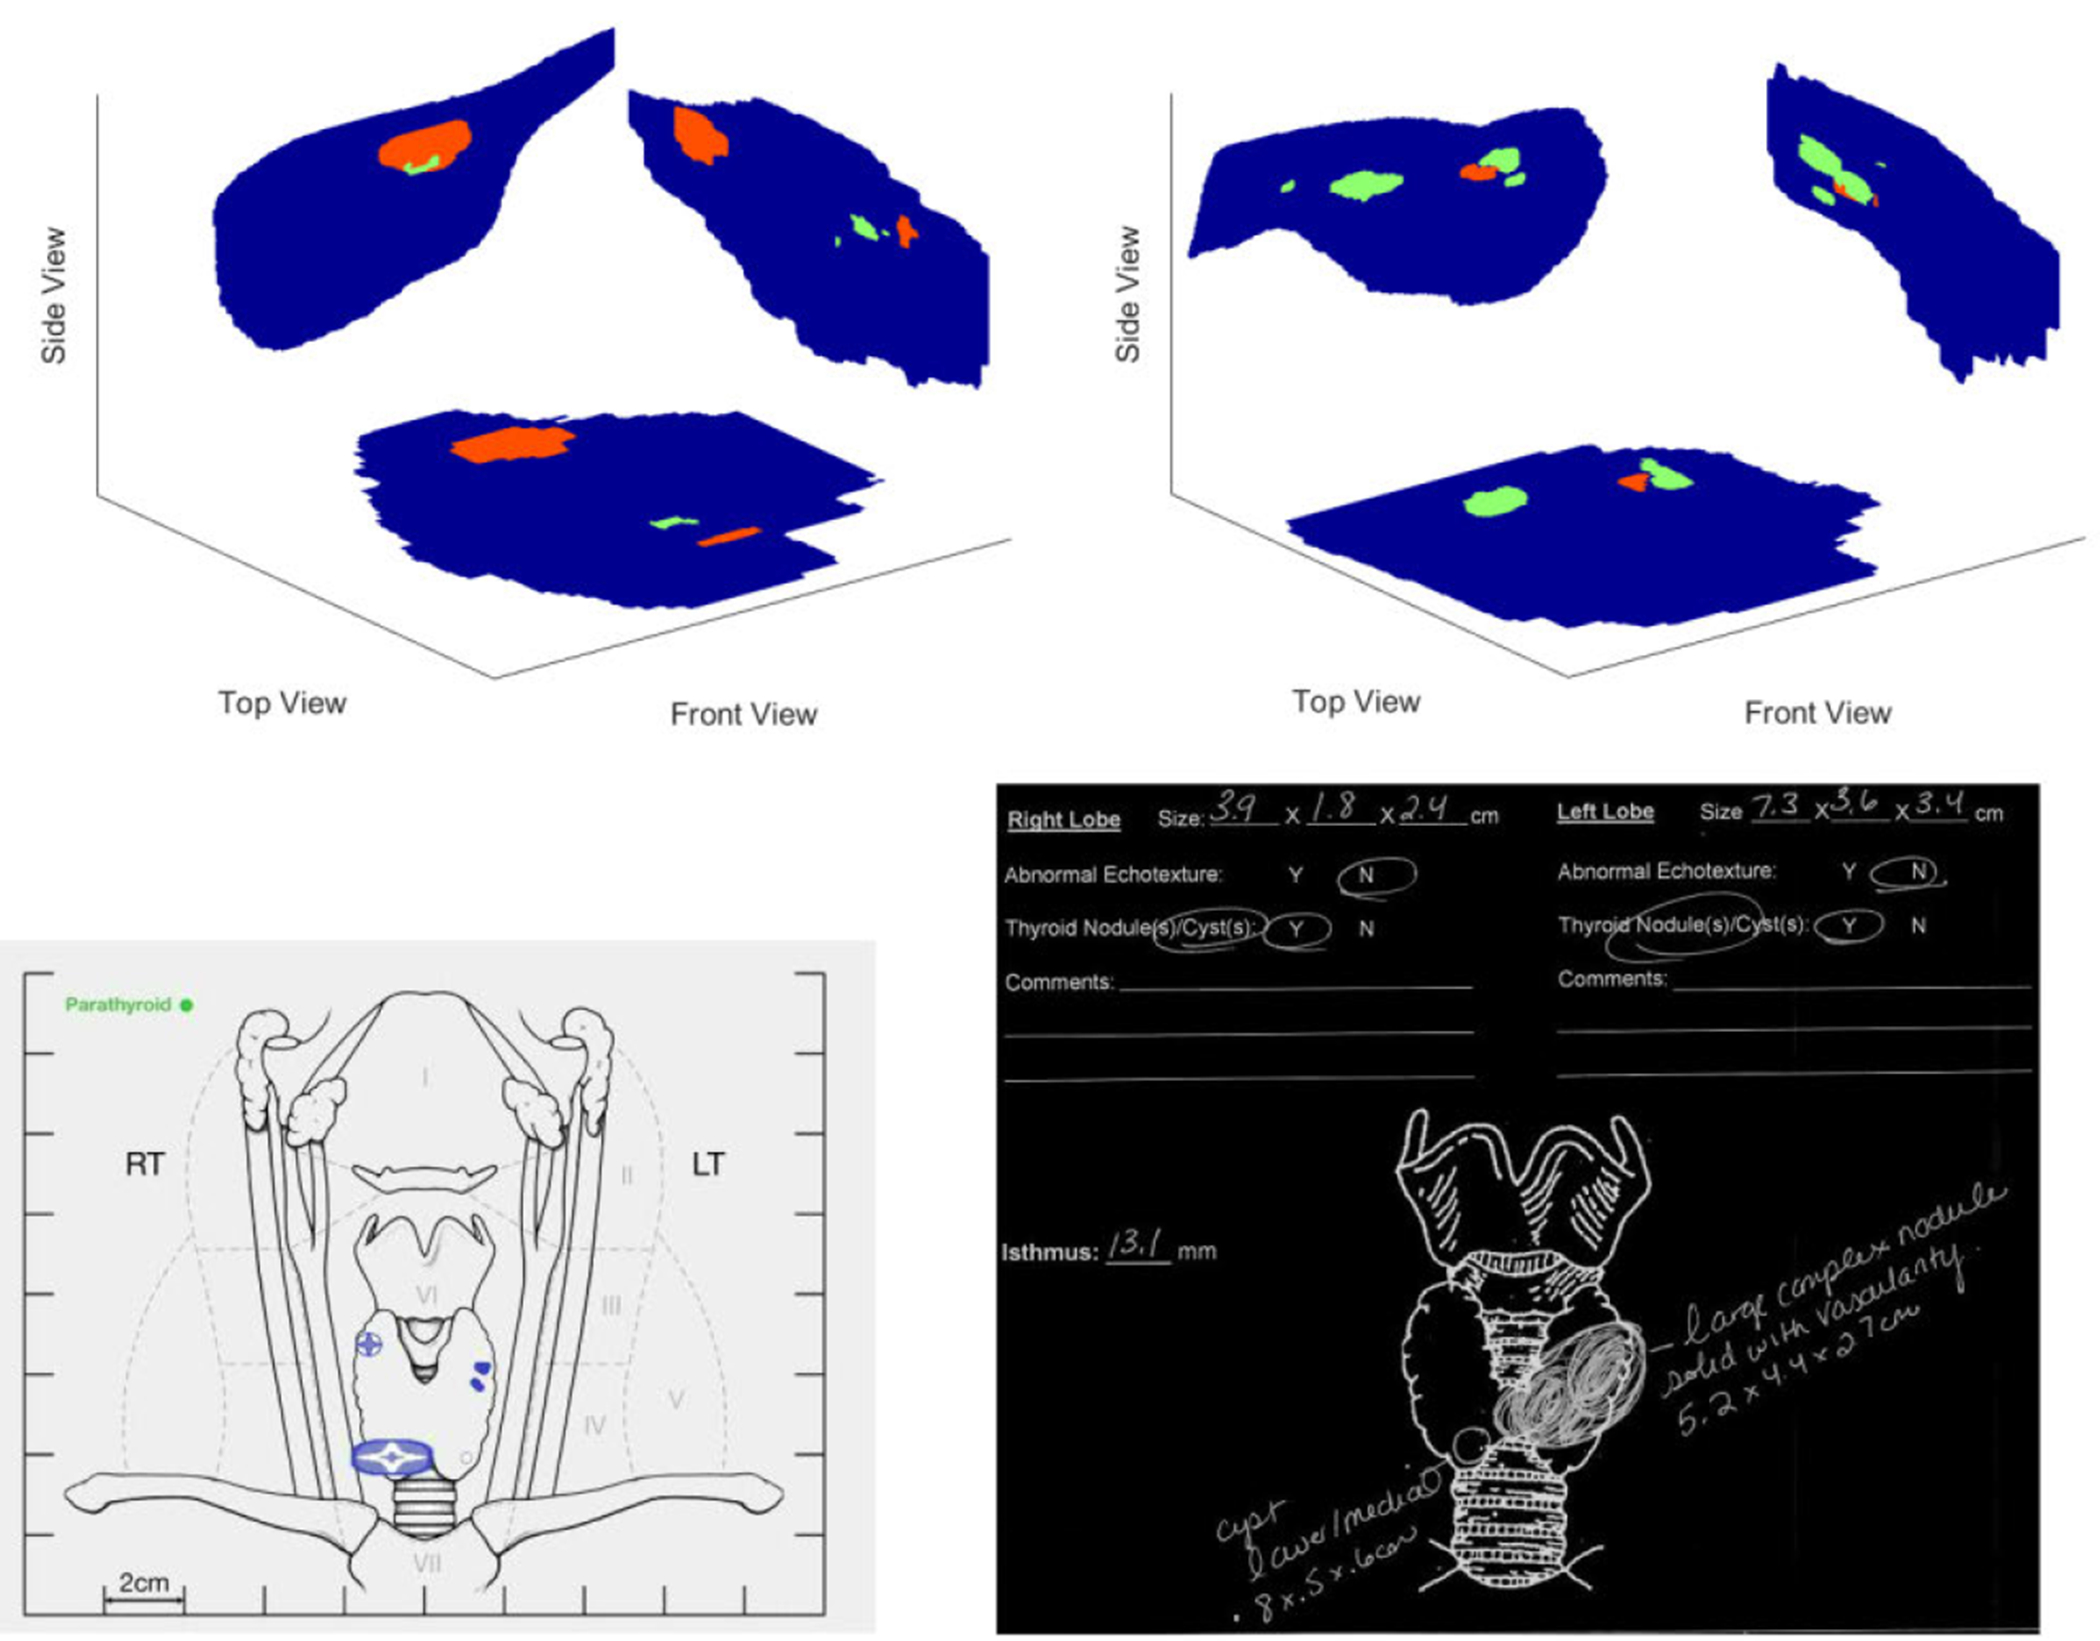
Examples of Comparison of automatically generated thyroid maps in multiple planes with thyroid maps generated by sonographers. In the generated maps blue represents the thyroid gland, red represents solid nodules and green represents cysts.
